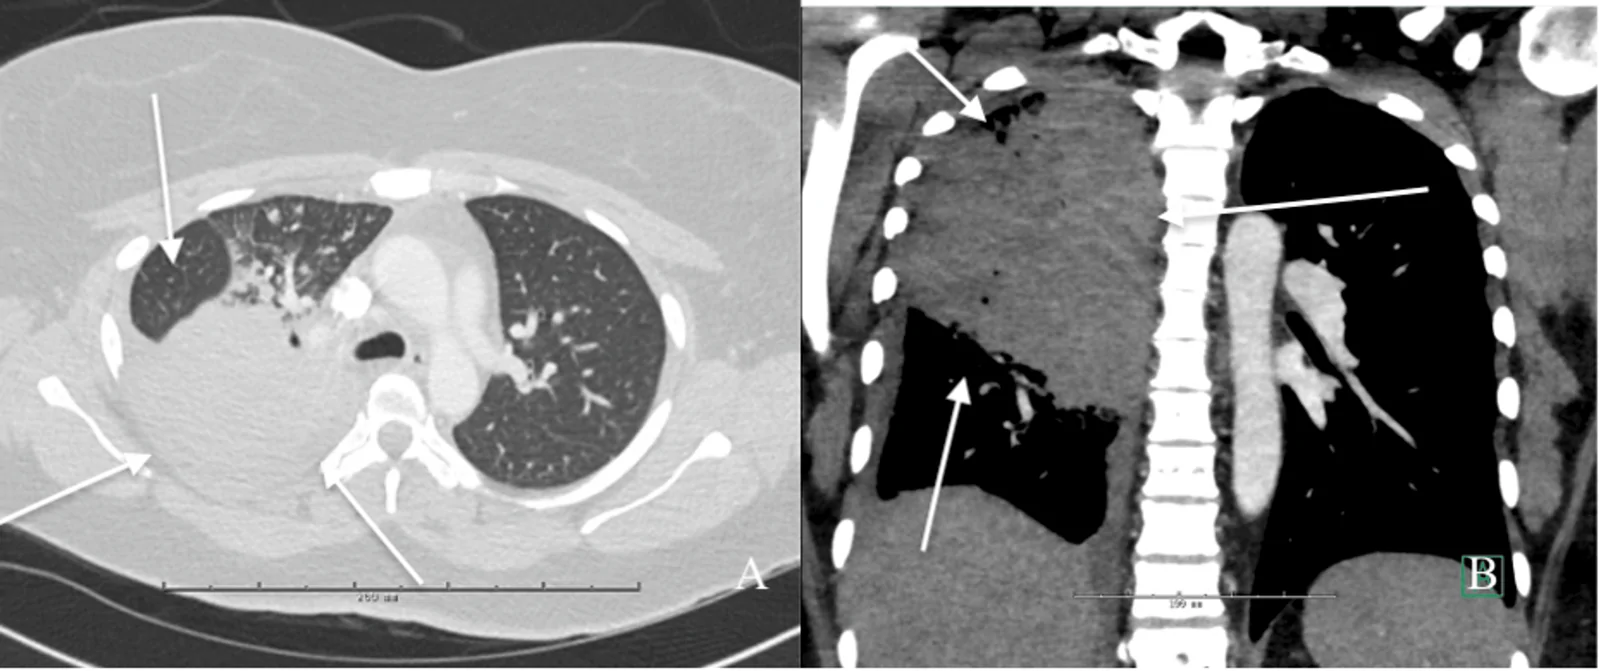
CT angiogram of the chest obtained during repeat presentation. A: axial view; B: coronal view demonstrating interval progression of right-sided pulmonary consolidation involving the upper and lower lobes with internal air bronchograms. Arrows point to the right lung consolidation that now involves both the upper and lower lobes.
